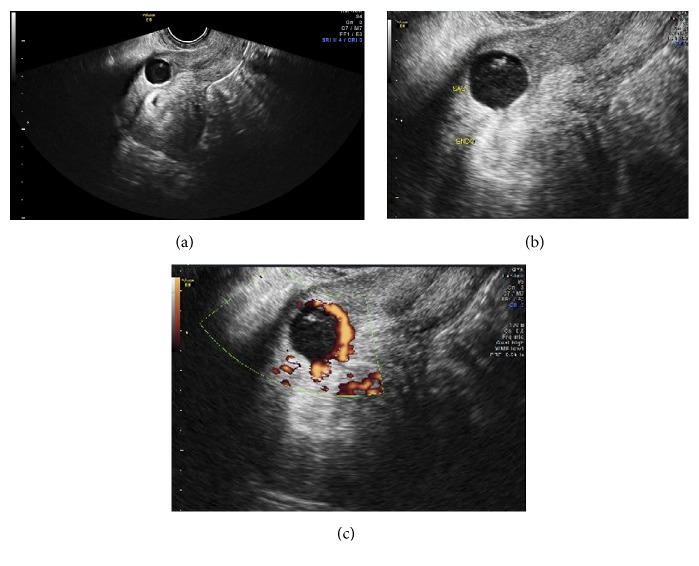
Transvaginal ultrasound showing (a) a cesarean scar ectopic pregnancy with gestational sac and yolk sac in the prior cesarean scar; (b) delineation of ectopic pregnancy and empty endometrial lining; (c) trophoblastic/placental blood flow using color Doppler.
